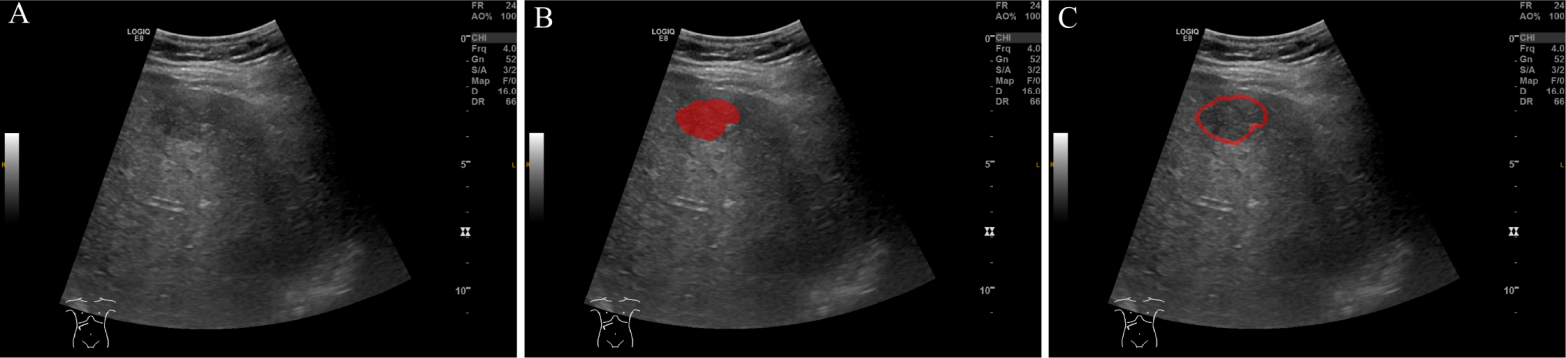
ROI (Region of Interest) delineation on ultrasound images. (A) Original ultrasound image. (B) ROI delineation of the tumor region (in red). (C) ROI delineation including the peritumoral region (in red), encompassing the area surrounding the tumor.
